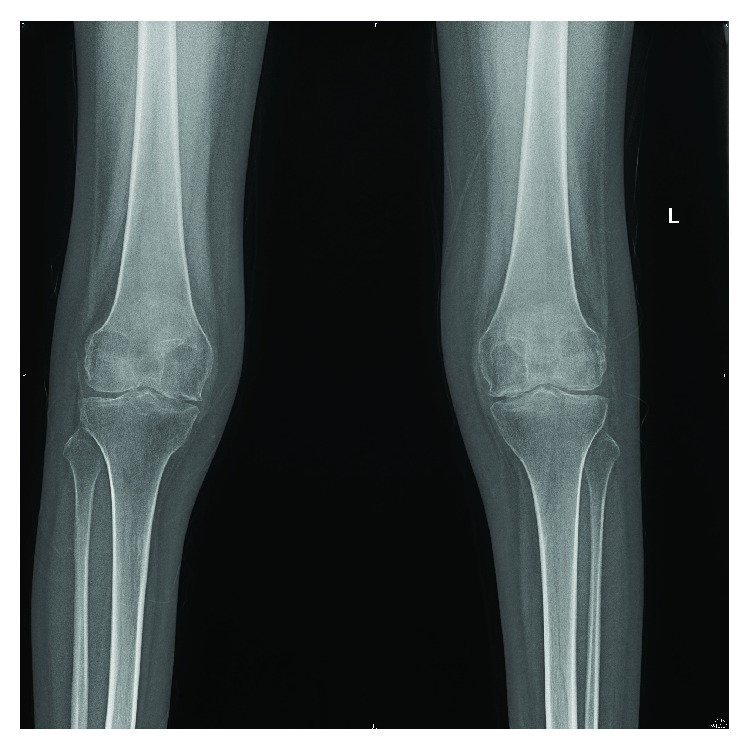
Plain knee radiographs at age 19.
